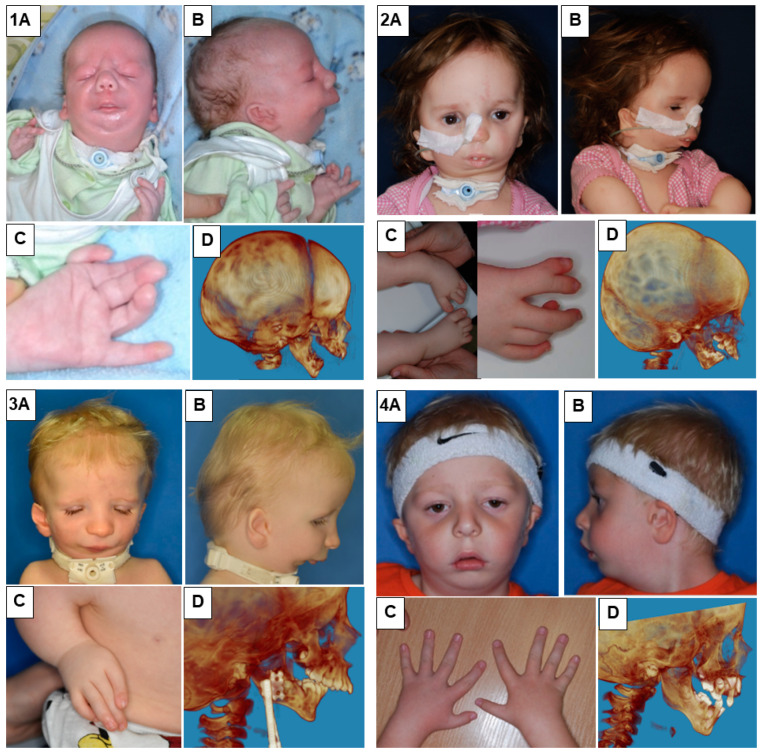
Patients with Nager syndrome: (1) newborn, (2) three years of age, (3) two years of age, and (4) five years of age. (A): front view. (B): lateral view. (C): (1) view of the hand with a vestigial undeveloped thumb, (2) the foot and lower leg with talipes equinovarus (equinus foot) and with abnormal toe structure, (3) the hand with a misaligned and undeveloped thumb as well as characteristic position of the arm due to contracture of the elbow joints and underdevelopment of the bones, and (4) patient with all five fingers on each hand. (D): 3D reconstruction of a CT image lateral projection.
